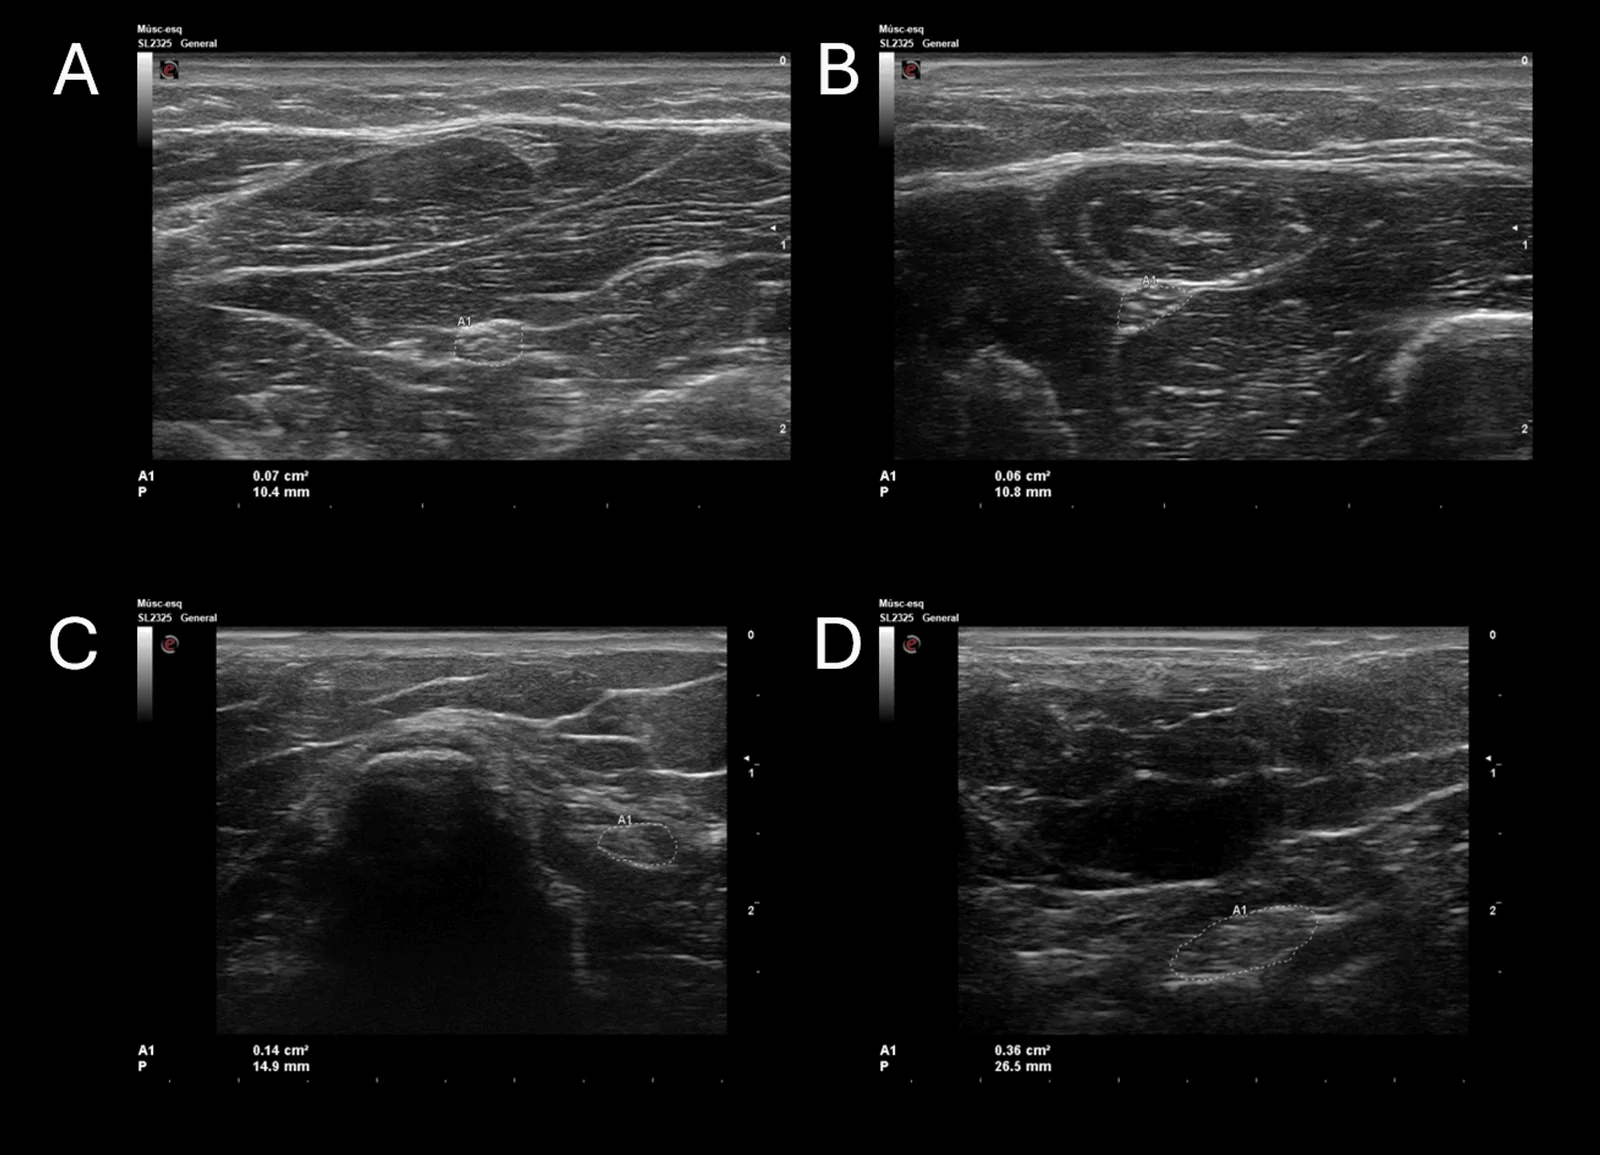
Normal neuromuscular ultrasound of peripheral nerves and adjacent muscles. Neuromuscular ultrasound showing normal nerve morphology and cross-sectional area of the right median nerve in the forearm (A), right ulnar nerve in the forearm (B), right fibular motor nerve at the knee (C), and right tibial nerve at the knee (D). Adjacent muscles showed no sonographic neuropathic or ALS-compatible changes, including no abnormal echogenicity, atrophy, or fasciculations. ALS: Amyotrophic lateral sclerosis.
